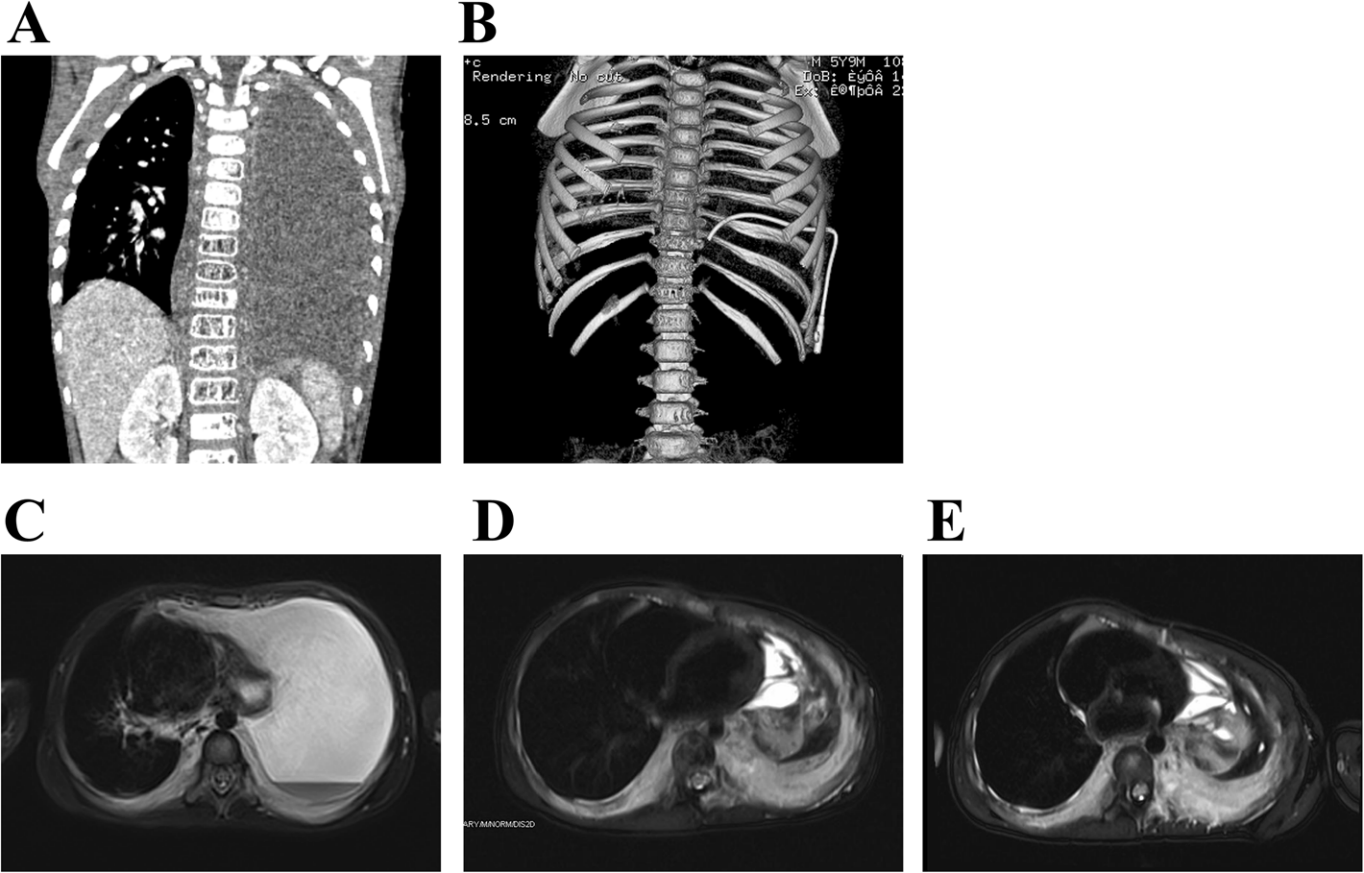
Treatment response in patient with KLA (Patient #1). a At the initial presentation of respiratory distress, coronal enhanced CT scan revealed massive pleural effusion almost entirely filling the left hemithorax with complete atelectasis of the left lung and mediastinal shift to the right. The lesions involved the vertebral bodies of T8-T12 and multiple ribs (b). Horizontal T2-weighted MRI revealed pleural thickening and effusion, heterogeneously increased T2 signal intensity along the right tracheobronchial tree, and infiltrative, hyperintense, posterior mediastinal soft tissue masses (c). The patient was treated with vincristine for 12 months. However, the response was suboptimal. The lesion became progressively static in size (d). Treatment with sirolimus and prednisolone was then initiated. After 8 weeks of combination treatment with sirolimus and prednisolone, followed by 10 months of sirolimus monotherapy, the lesions became more prominent (e)
